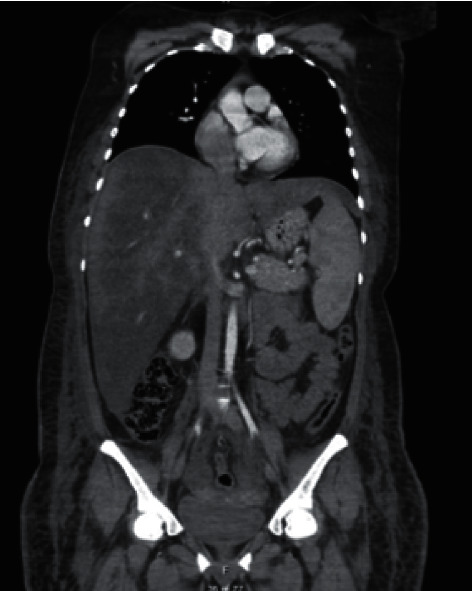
Computed tomography that shows massive hepatomegaly (29 cm) and splenomegaly (16 cm).
